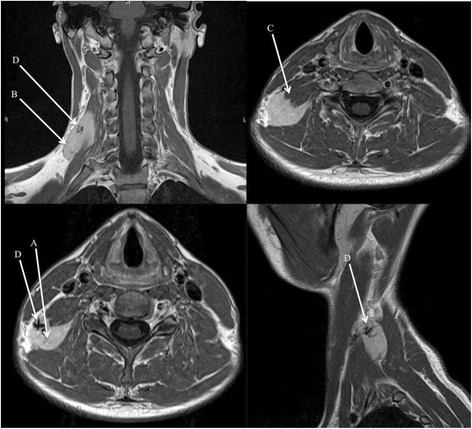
MRI of the hibernoma. The MRI (T1) revealed a 38 mm along the axis mass of the posterior cervical triangle with septa > 2 mm (a), nodular structures (b), muscular invasion (c), and a high vascularization (d).
